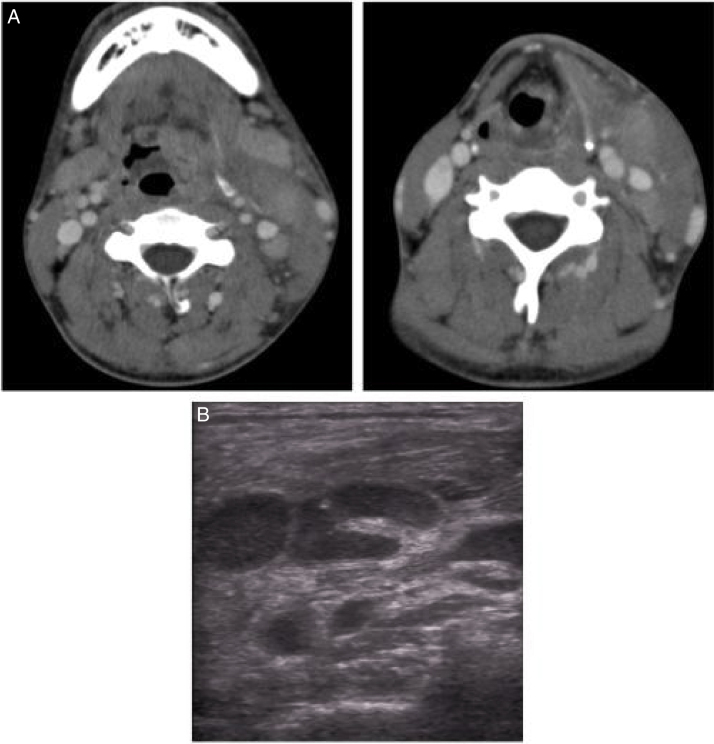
Cervical ultrasonic examination and enhanced computerized tomography (A, B). (A) Ultrasonic examination shows swellings of cervical lymph nodes. (B) Enhanced computed tomography shows swellings of cervical lymph nodes and edema of soft tissue and sternocleidomastoid muscle.
